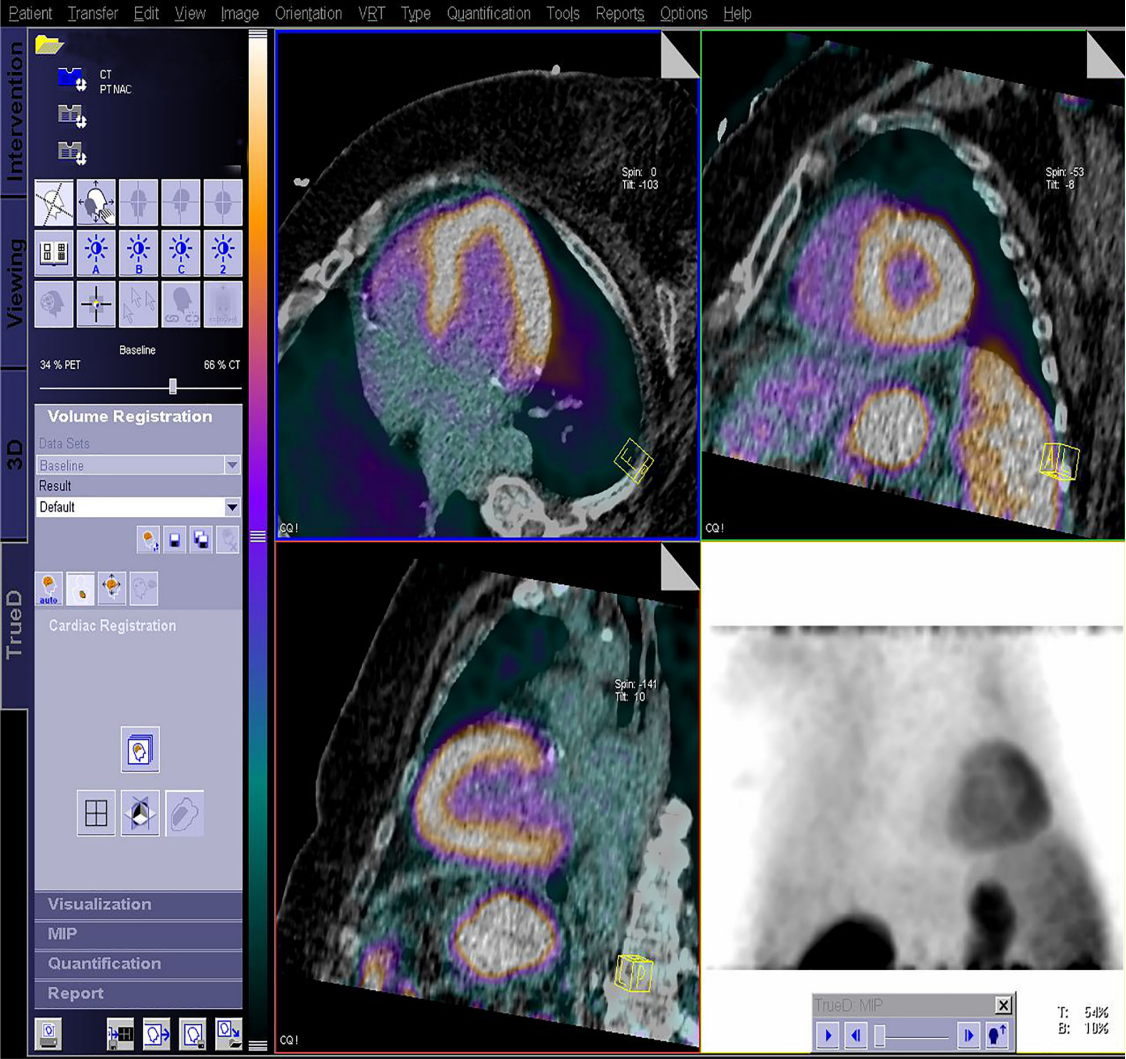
SMART Auto Cardiac Registration automatically aligns the non-attenuation-corrected (NAC) PET with the CT data in order to ensure optimal AC. The fused data is presented in transverse, coronal, and sagittal views. Data courtesy of University of Michigan, Ann Arbor, USA
